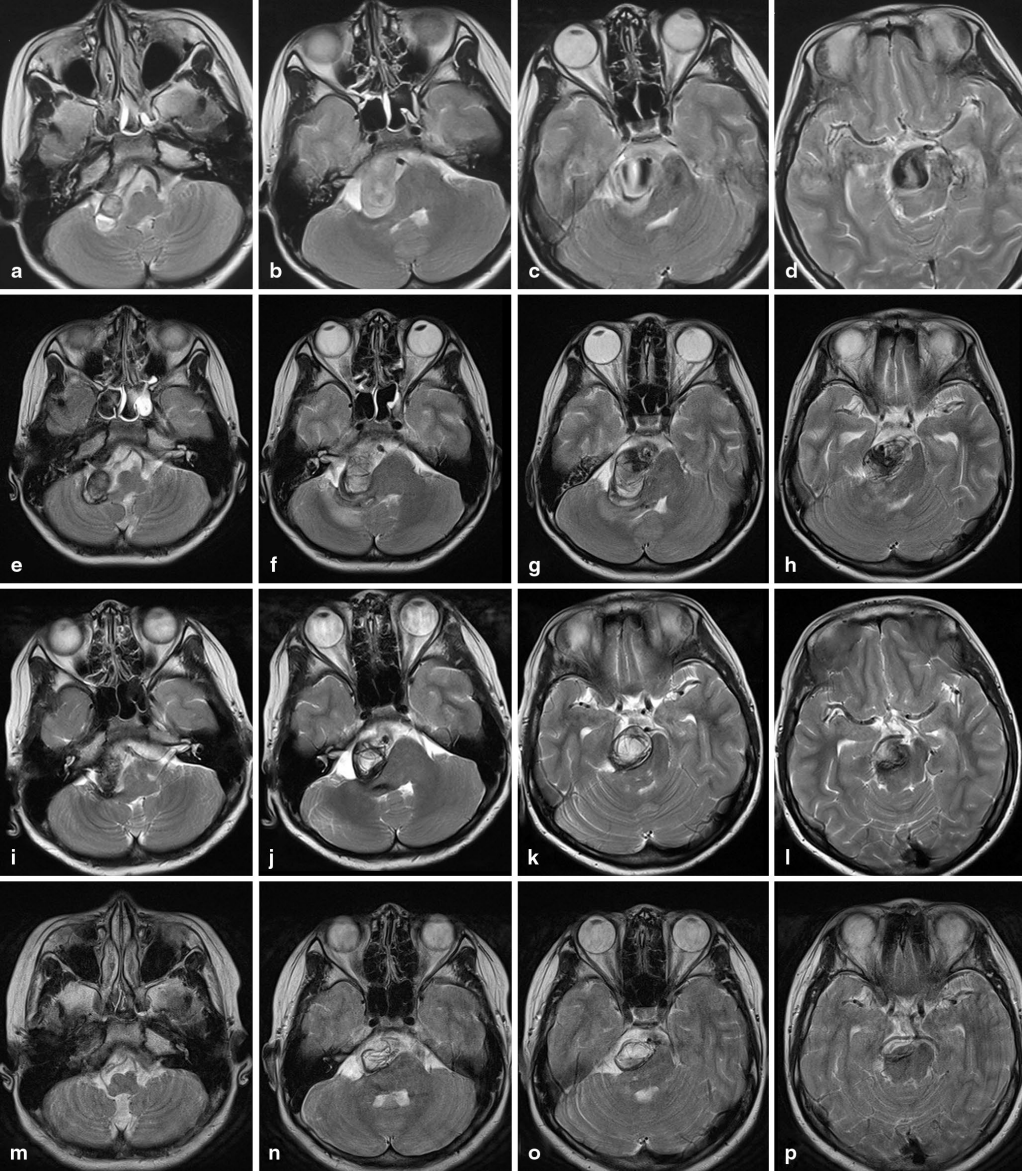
a–d The magnetic resonance imaging (MRI) T2 revealed a partially thrombosed giant aneurysm of the basilar artery trunk before treatment. e–h Follow-up MR confirmed the thrombosis of the aneurysm with an increase in the mass effect on the brainstem in the next week after treatment. i–l The 3-month MRI revealed significant reduction in the size of the aneurysm and in the mass effect on the brain stem. m–p The 23-month MRI showed further shrinkage of the aneurysm
